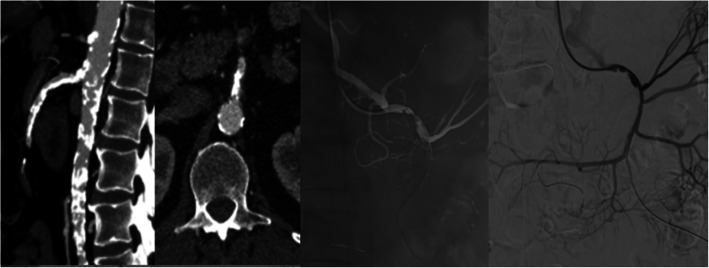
CTA revealed highly calcified stenosis in the proximal segment of SMA. Selective brachial access angiography confirmed severe SMA stenosis
